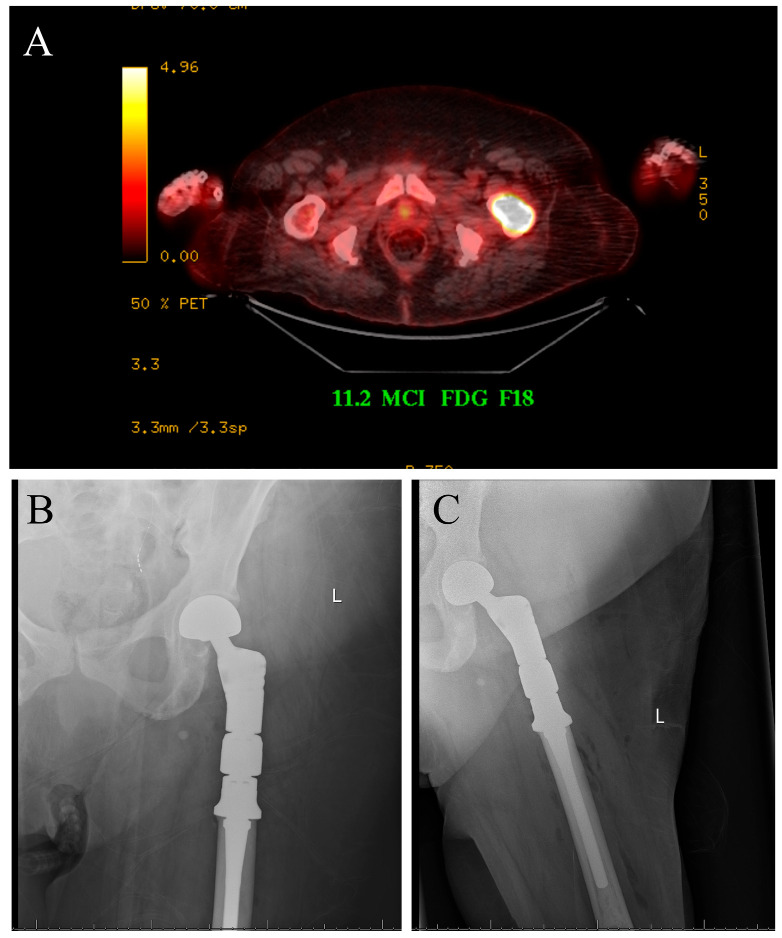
Seventy-five-year-old female with newly diagnosed breast cancer and pain with weight-bearing on the left hip. (A) Axial cut PET-CT showing solitary bone lesion biopsy proven breast cancer. (B,C) Patient underwent resection of left proximal femur and reconstruction with proximal femur megaprosthesis.
